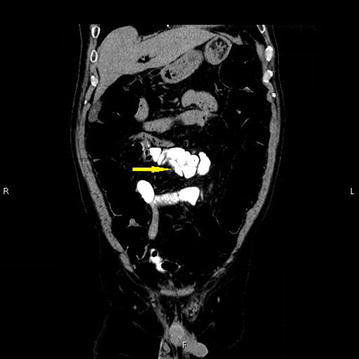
Abdominal CT enteroclysis image. CT enteroclysis was performed immediately after DBE enteroclysis in order to confirm the shape and size. There were no malignant findings in the duplication. The arrow shows the duplication
